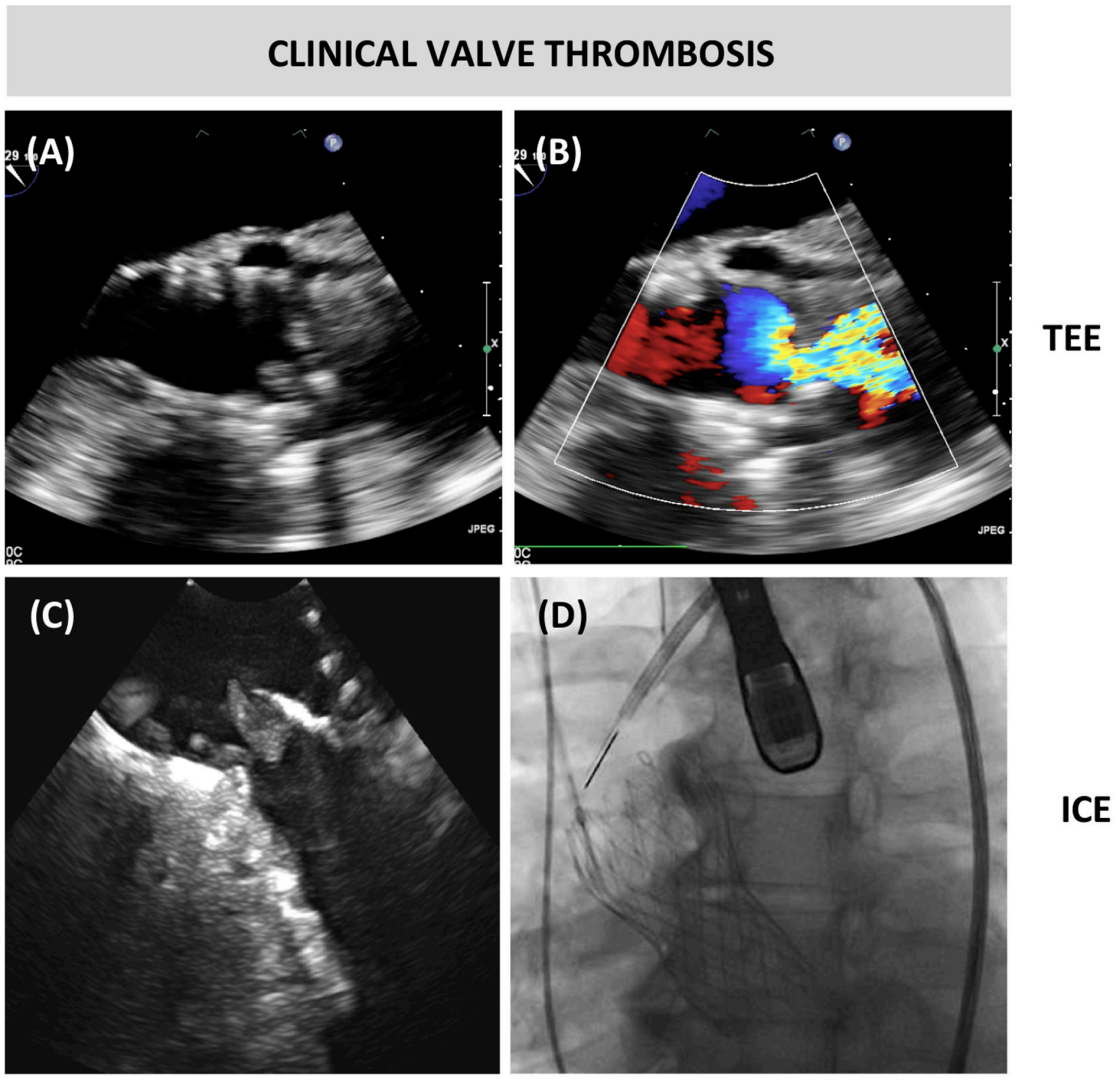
Clinical valve thrombosis. (A,B) Transesophageal echocardiography (TEE) showing thrombosis and turbulent color flow over a bioprosthetic aortic valve in a patient who underwent transcatheter aortic valve replacement 6 years earlier. The patient presented with dyspnea NYHA class 3b and echocardiography revealed a mean transvalvular gradient of 37 mmHg (C,D) The thrombotic mass at the prosthetic leaflets was confirmed by intracardiac echocardiography from the ascending aorta.
